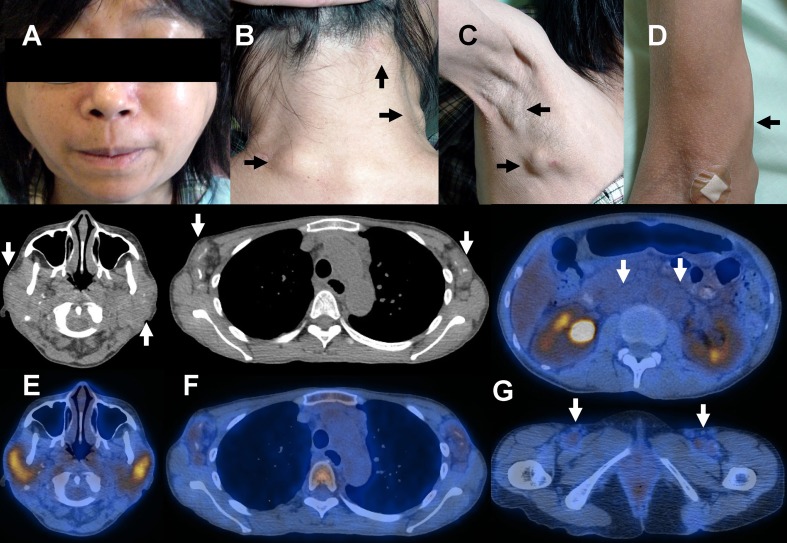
Patient with marked sicca symptoms. Bilateral parotid enlargement was found (a). There was generalized lymphadenopathy affecting the head and neck (b, *arrows*), axillae (c, *arrows*), epitrochlear (d, *arrow*), and groin regions. FDG PET/CT showed calcified parotid glands (e, *arrows*) with mild FDG uptake. There was generalized lymphadenopathy showing fine specks of calcification (f, *arrows*, axillae), with minimal FDG uptake. There was also para-aortic and groin lymphadenoapthy (g, *arrows*)
